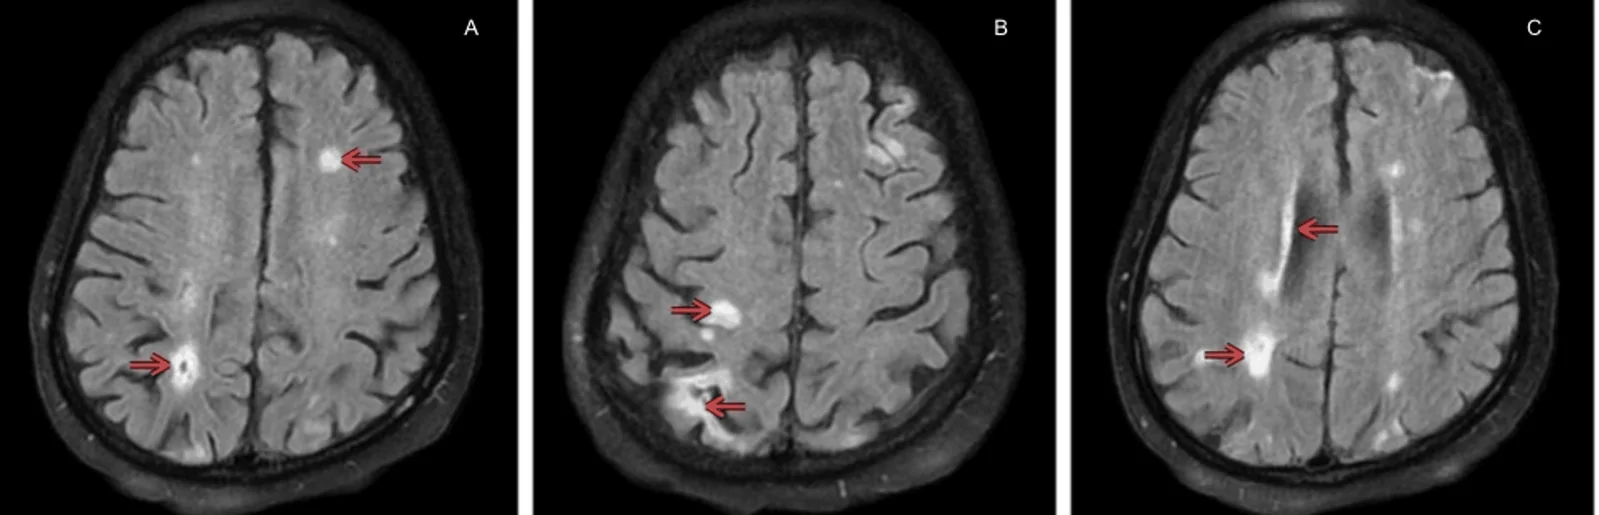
Brain MRI (second admission), fluid-attenuated inversion recovery imaging A-C: arrows indicate new bilateral foci consistent with interval recurrent thromboembolic infarction, evidence of ongoing embolization despite treatment, strongly implicating a persistent embolic source.
